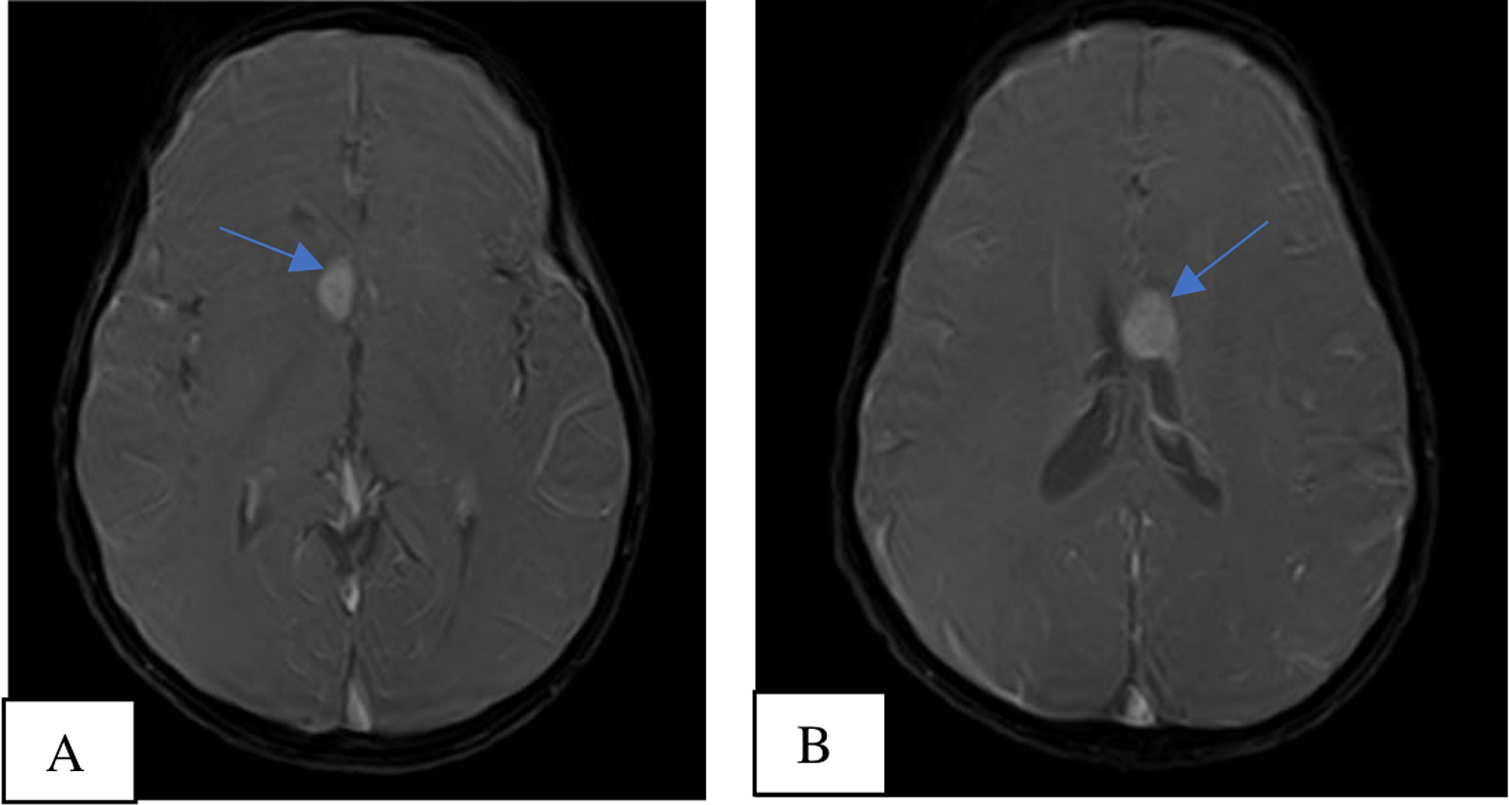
MRI, T1 axial FatSat contrast enhanced images at the level of the 3rd ventricle (3A) and body of the lateral ventricles (3B) showing the right and left Sub ependymal Giant Cell Astrocytomas (blue arrows).
